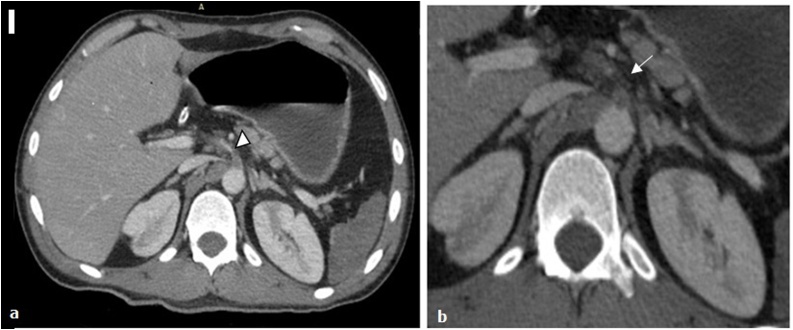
a: Axial CT showed an extended thrombosis (Arrowhead) of common celiacomesenteric trunk, the aorta is normal. b: A non-specific periarterial fat infiltration (Arrow) around this trunk was found.
